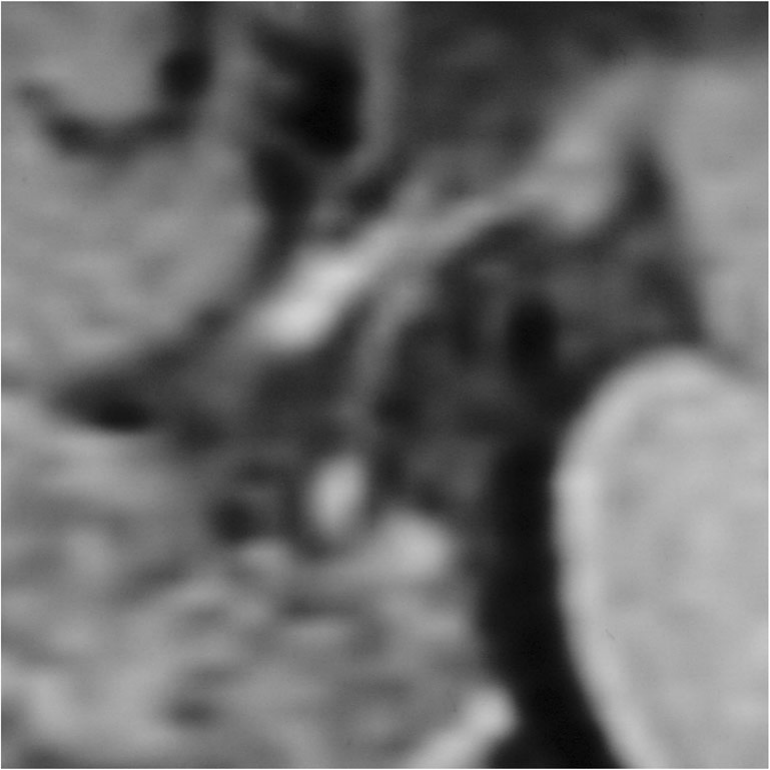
Agenesis of the anterior pituitary gland (adenohypophysis) in an infant with hypopituitarism. Sagittal T1-weighted imaging (T1WI) shows absence of the adenohypophisis. The stalk terminates into a bulbous neurohypophysis with high signal in the dorsal portion of the sella
